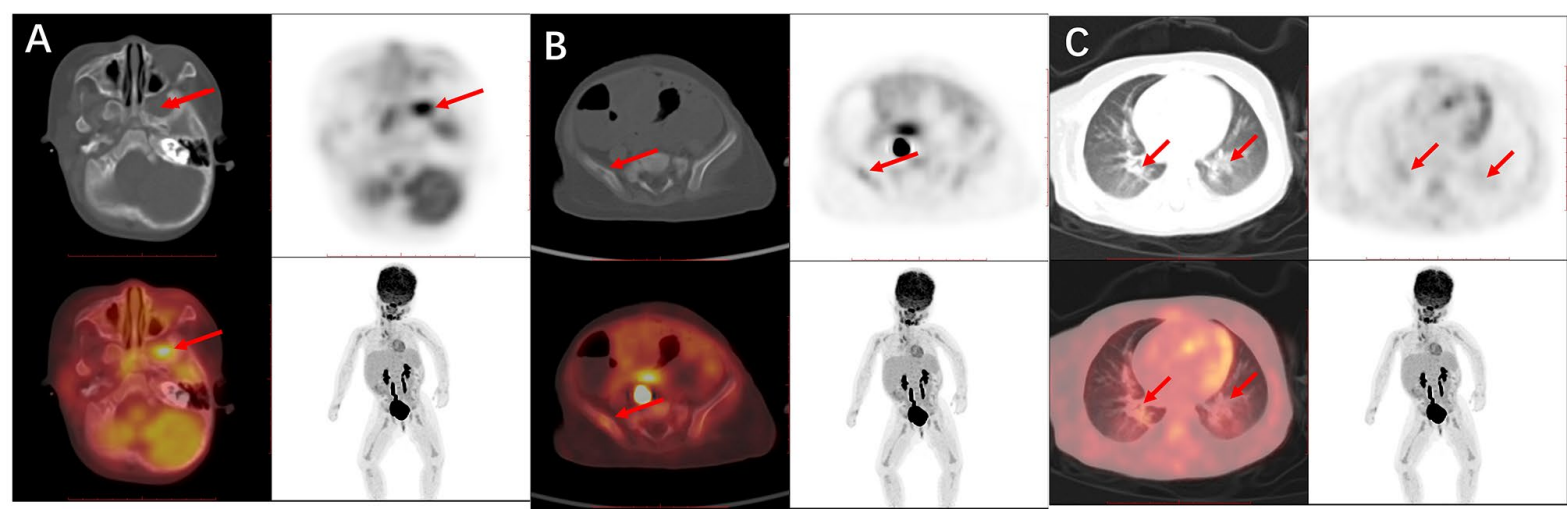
Positron emission tomography (PET)-CT image showing osteolytic lesions in the great wing of the sphenoid bone (A), the iliac bone (B) and pneumonia-like manifestations (C)
